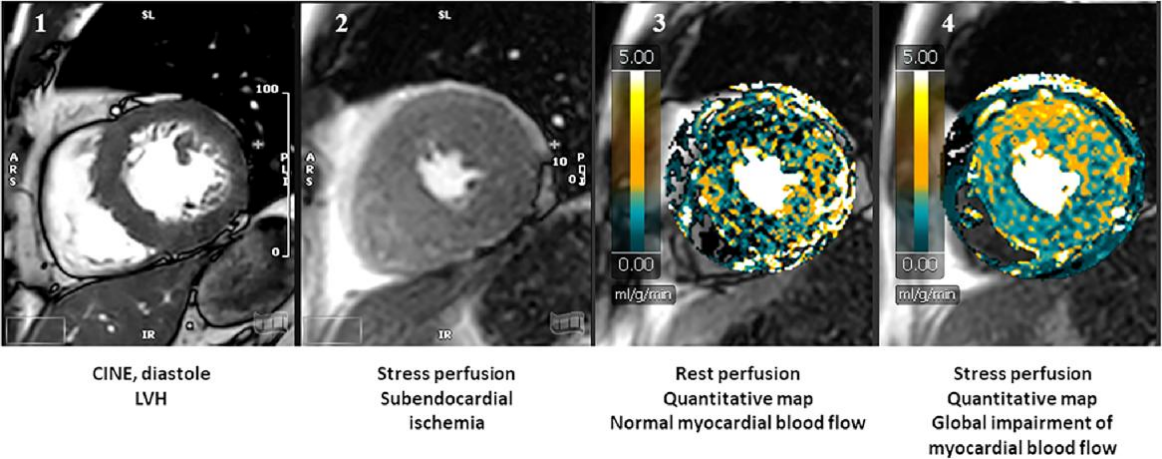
Example of HFpEF due to coronary microvascular dysfunction. Patient with heart failure symptoms and newly diagnosed left ventricular hypertrophy on echocardiography and CMR (panel 1). Panel 2 shows a thin circumferential subendocardial perfusion defect, suggestive for microvascular dysfunction. Microvascular dysfunction is clearly demonstrated by quantitative perfusion CMR (1.5 T): panel 3 shows normal perfusion at rest (green, rest myocardial blood flow 1.0 mL/g/min), while stress perfusion shows generalized impaired maximal perfusion (green-yellow; maximal myocardial blood flow 1.5 mL/g/min), resulting in a myocardial perfusion reserve of 1.5 (normal > 2.4).
